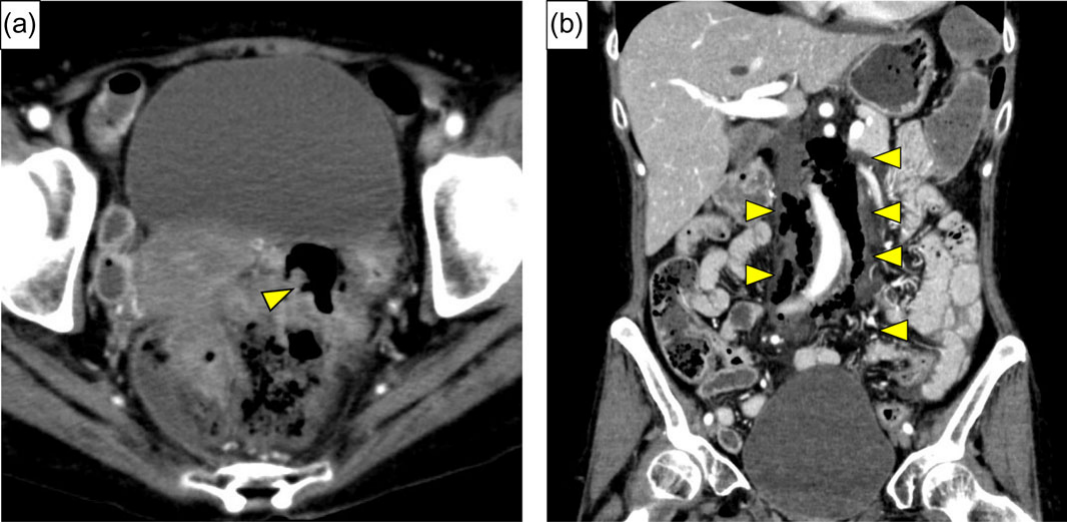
(a) Enhanced abdominal CT showed free air around the rectum (yellow arrow), and strongly suggested perforation of the rectum. (b) Massive continuous pneumoretroperitoneum from rectum was revealed (yellow arrows).
